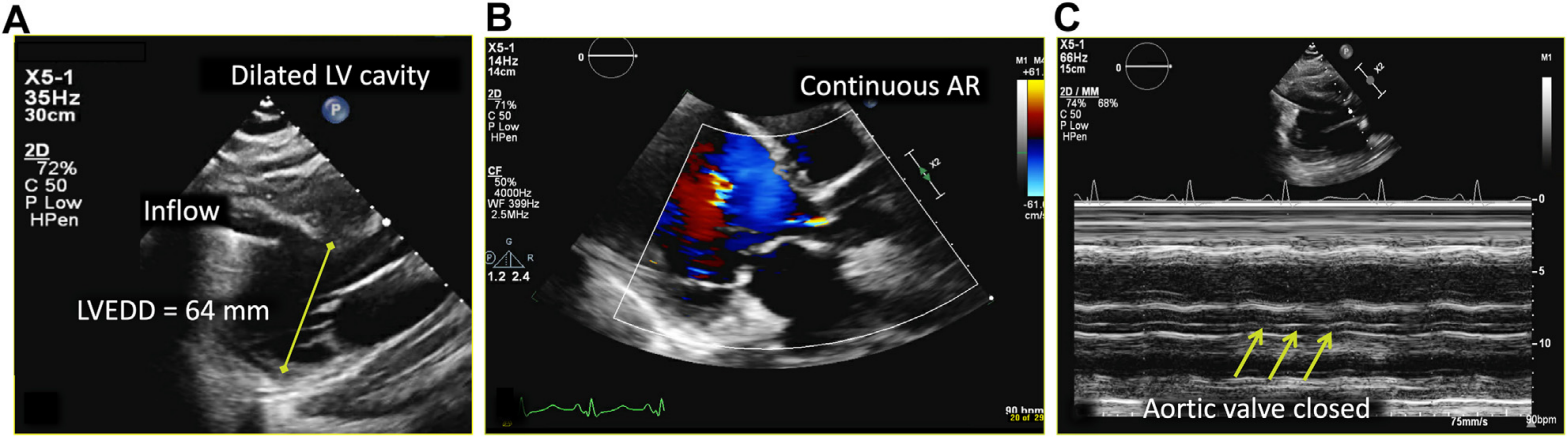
Two-dimensional TTE at admission, parasternal long-axis view without (A) and with (B) color flow Doppler and M-mode (C), demonstrates a dilated left ventricular (LV) cavity (LV end-diastolic diameter [LVEDD] 64 mm), adequately positioned inflow LVAD cannula, continuous aortic regurgitation (AR), and a persistently closed aortic valve.
